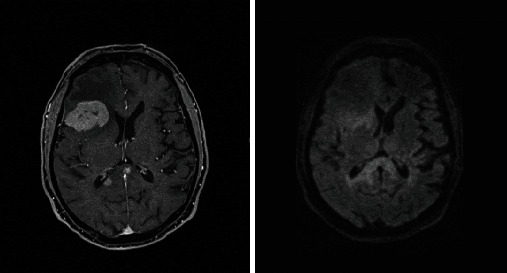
Multiple (at least 6) intra-axial brain masses with the largest in the right frontal lobe. Radiographic differential diagnosis: multifocal lymphoma and intracranial metastasis.
